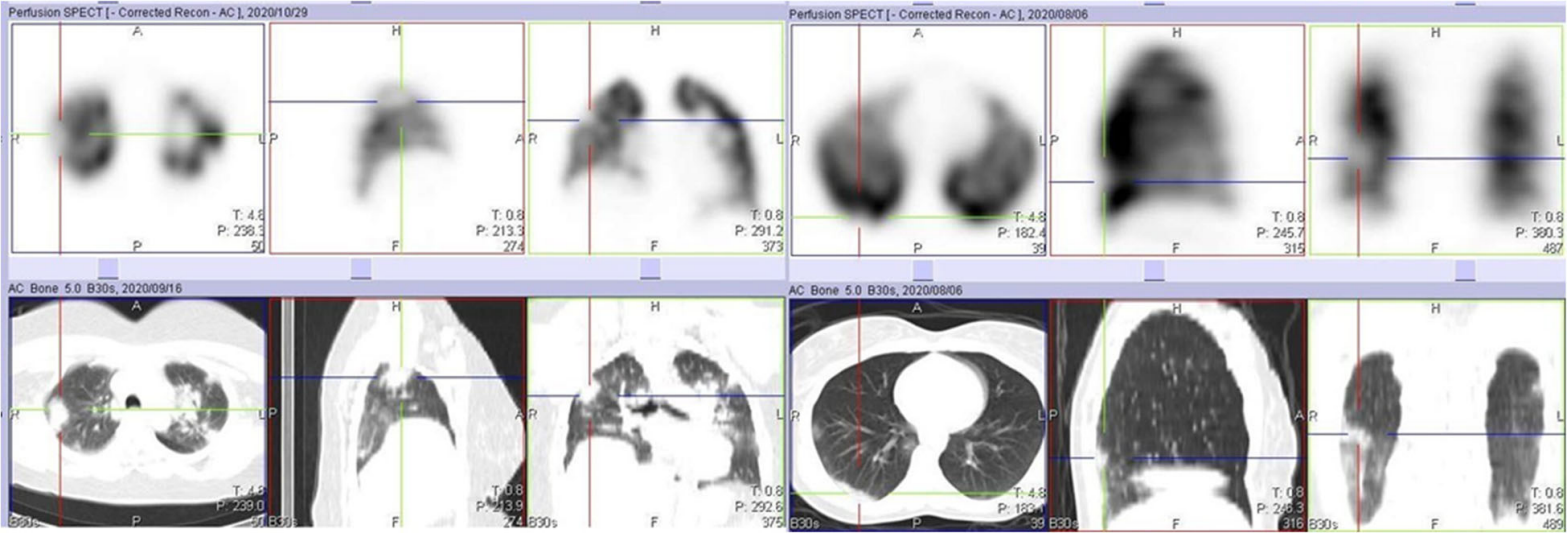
Perfusion only SPECT/CT images of two cases showing perfusion defects in areas with COVID pneumonia
